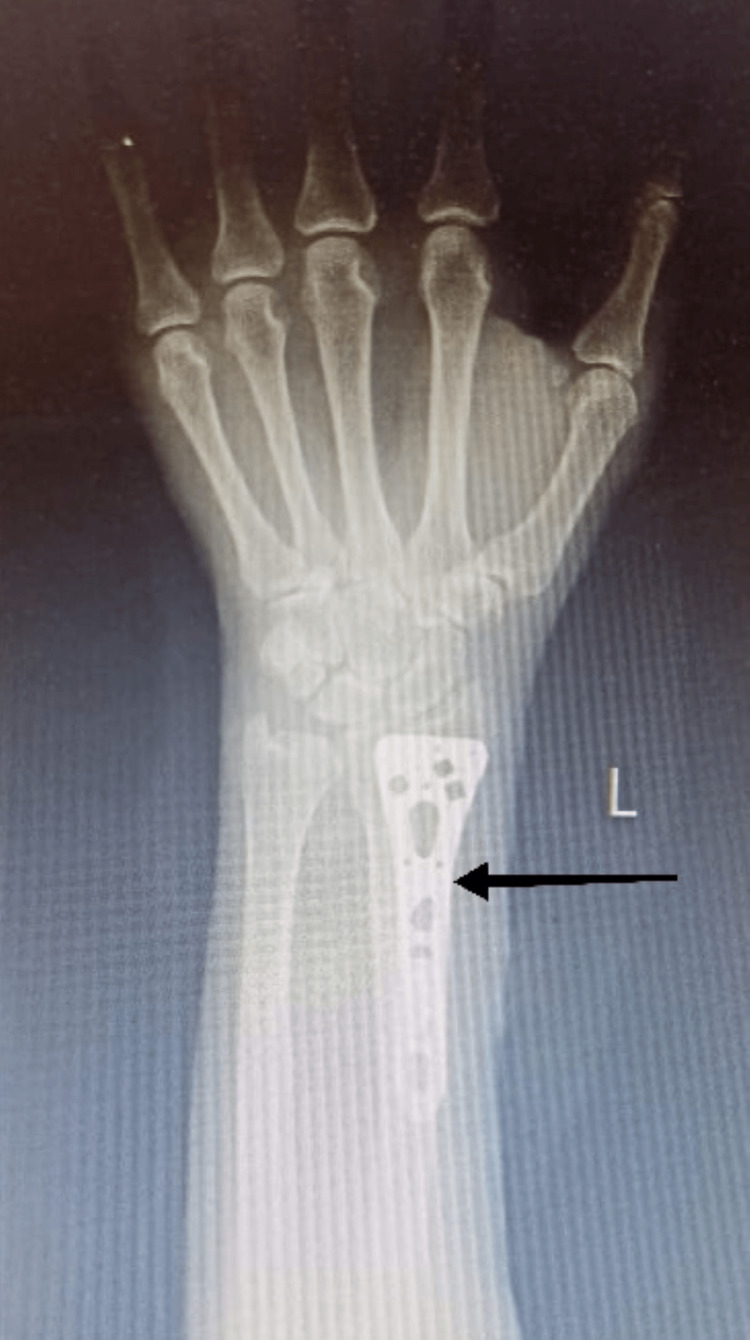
X-ray of patient’s left wrist postoperatively.
